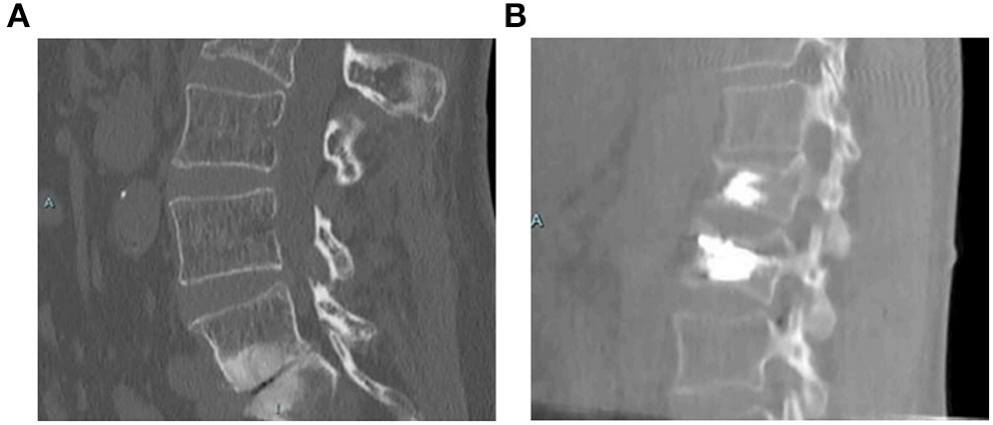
Treatment with PKP (47). A 58-year-old female with a non-specified mitochondrial pathology and a history of L1 vertebral fracture treated with kyphoplasty. She presented with pain in the lumbar region after a fall. She was treated with PKP, and demonstrated an unremarkable postoperative course. (A) Computed tomography scan showing a L2 fracture with a deformity in both the sagittal and coronal planes. (B) Postoperative scan showing correction in both the sagittal and coronal planes. PKP, percutaneous balloon kyphoplasty.
